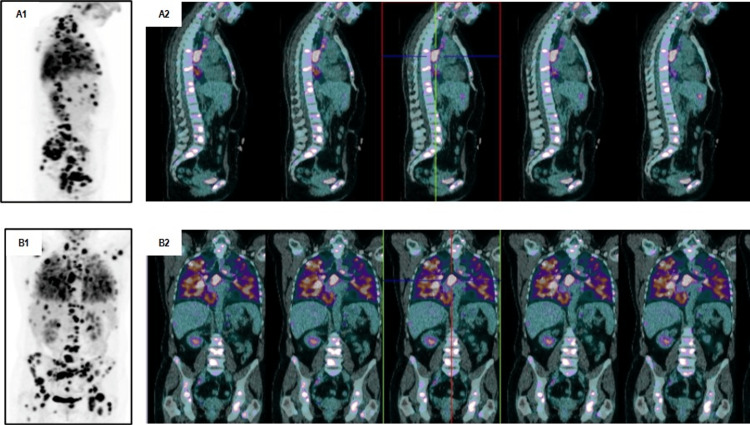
Positron-emission tomography-computed tomography (PET-CT) scan images demonstrating extensive metastatic disease at diagnosis with high maximum standardized uptake value (SUV) in lungs, lymph nodes (supraclavicular, paratracheal, prevascular, aortopulmonary, subcarinal and hilar bilateral), liver, left suprarenal gland and bone (all spine, sacred bone, pelvis, sternum, scapulas, right clavicle, ribs, humerus, and femurs). A1) maximum-intensity-projection images on sagittal plane; A2) PET/CT sagittal view; B1) maximum-intensity-projection images on coronal plane; B2) PET/CT coronal view.
